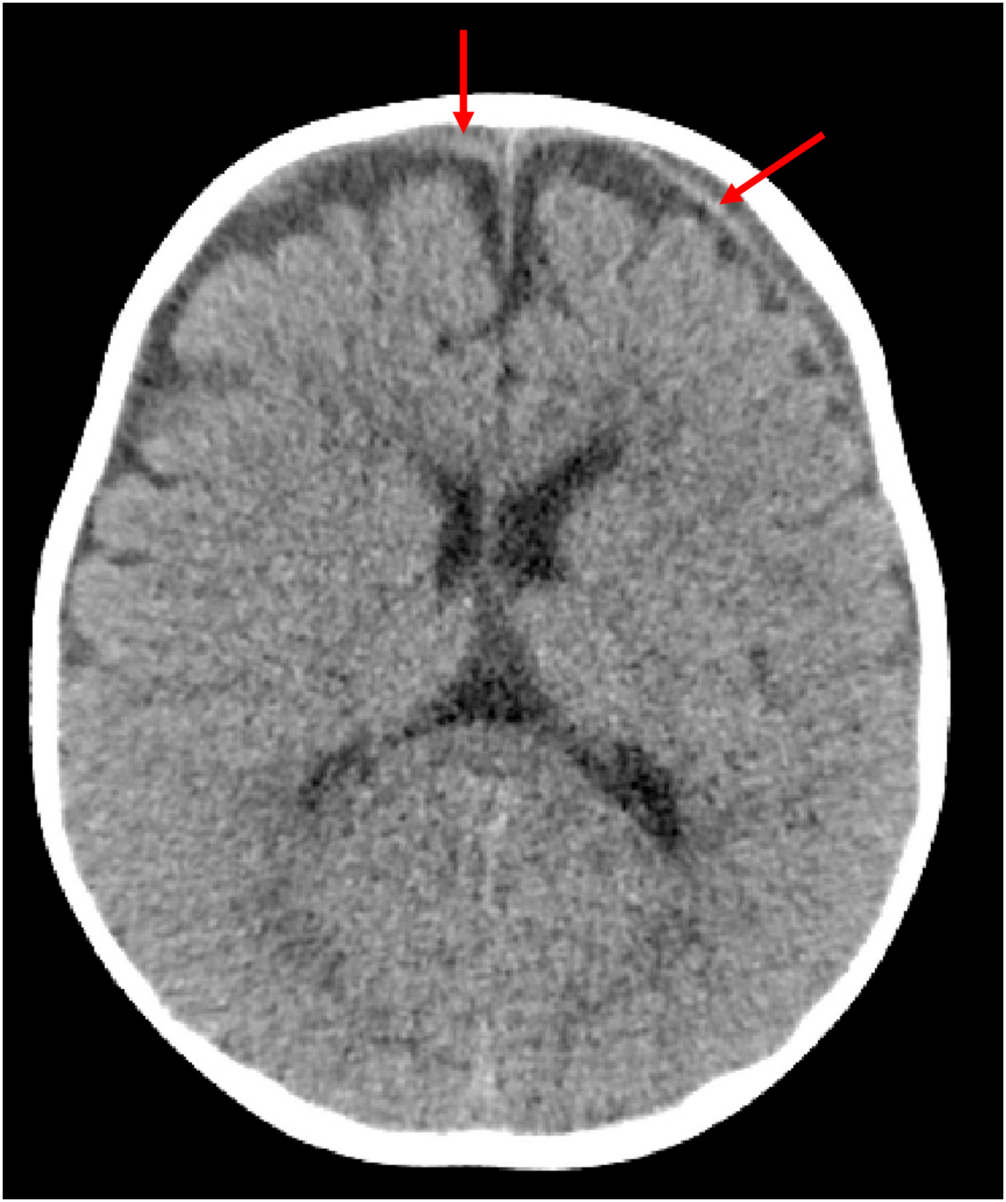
Axial image non‐contrast CT brain showing hyperdense (acute) linear subdural collection on the left with associated hypodense subdural fluid (chronic collection). There is a smaller hyperdense collection on the right.
